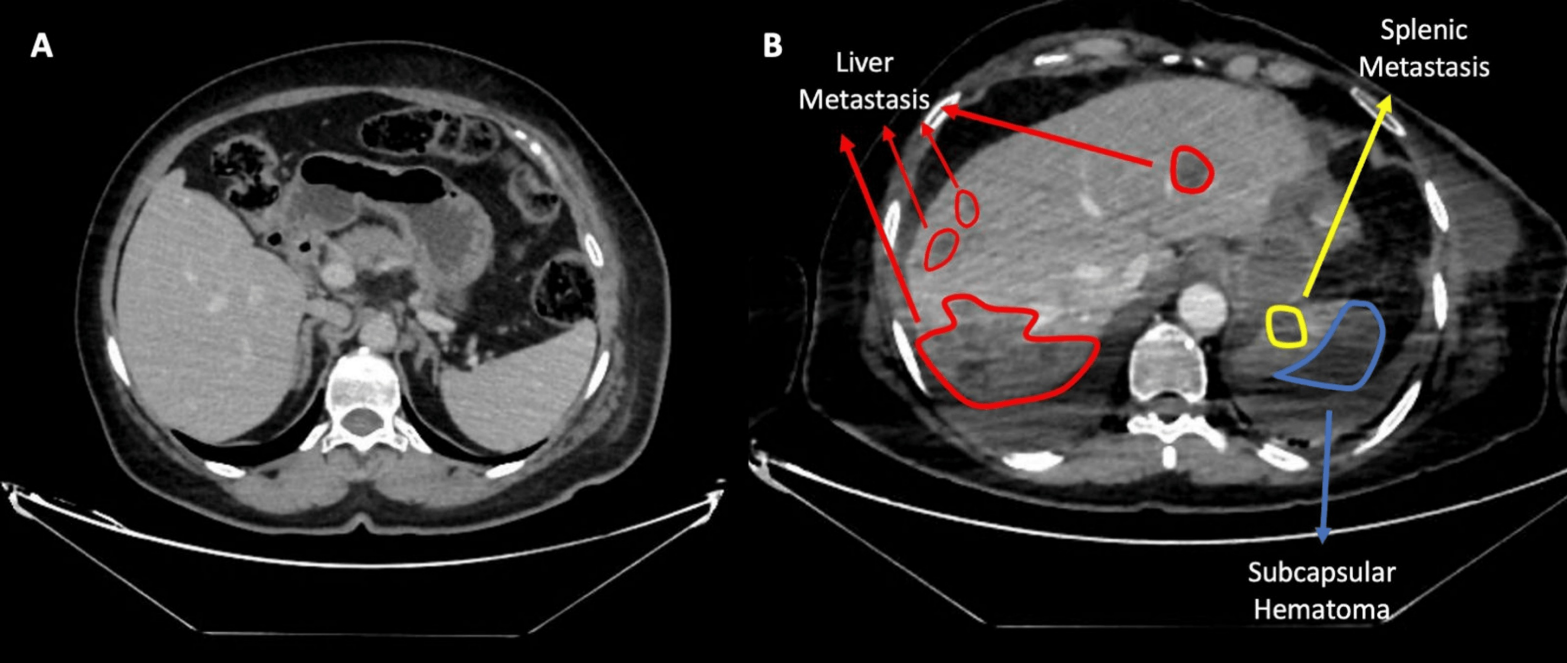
Serial post-contrast CT images demonstrating interval development of hepatic and splenic metastases with associated subcapsular hematoma A) Post-contrast axial CT image showing no metastatic lesions in the liver or spleen B) Post-contrast axial image obtained three months later demonstrating metastatic lesions in the liver and spleen, accompanied by the development of a subcapsular hematoma
